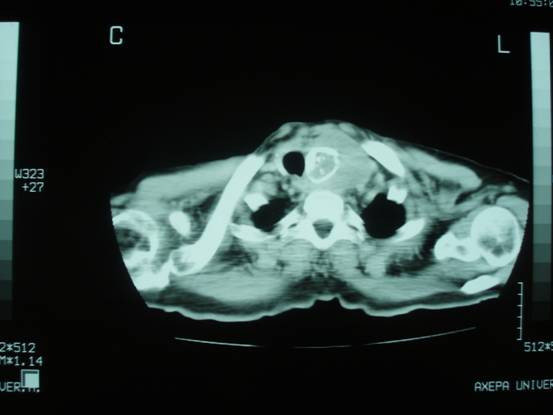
Computed tomography scan of the cervical neck.
